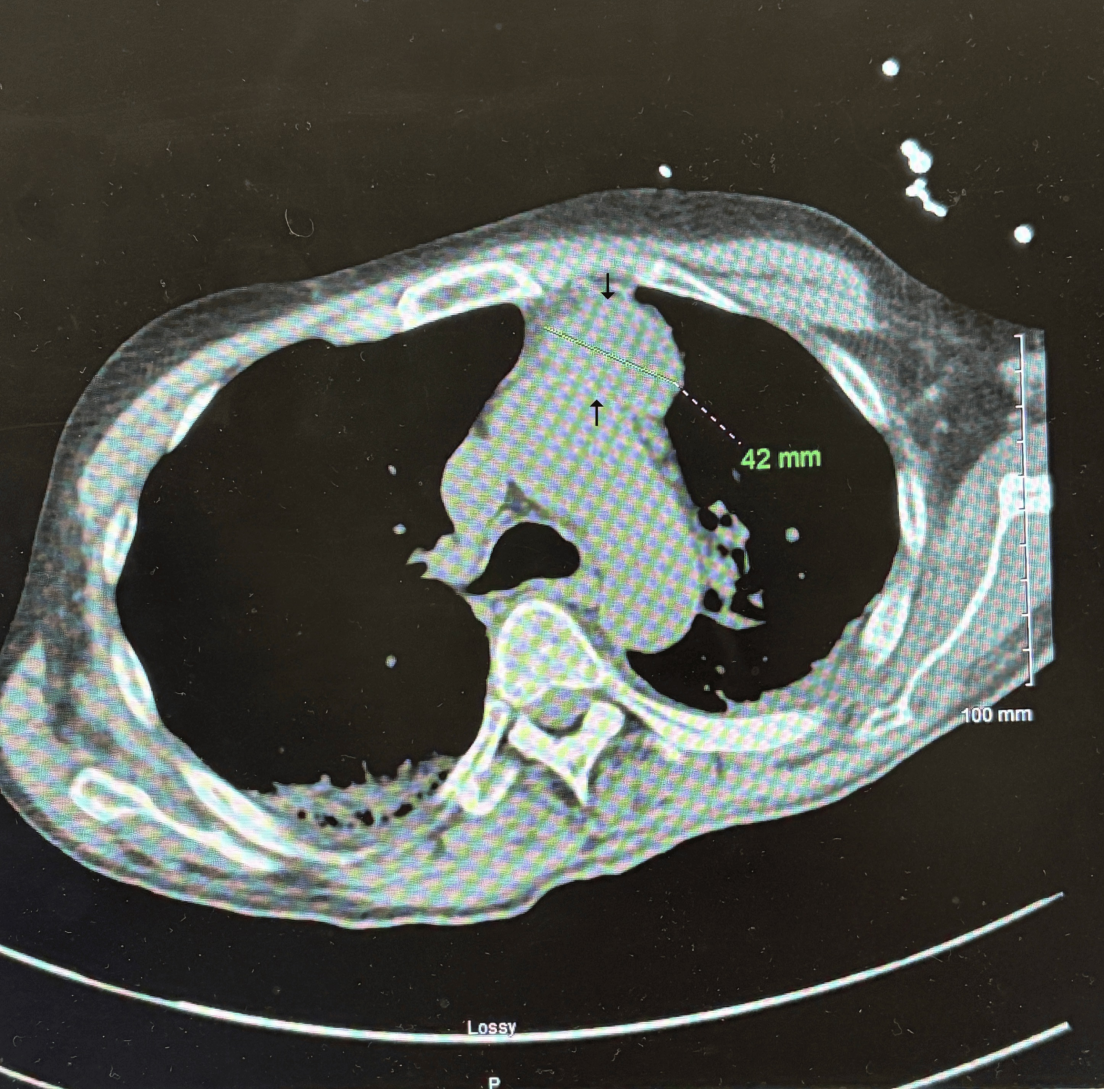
Chest CT revealing a large anterior mediastinal mass
